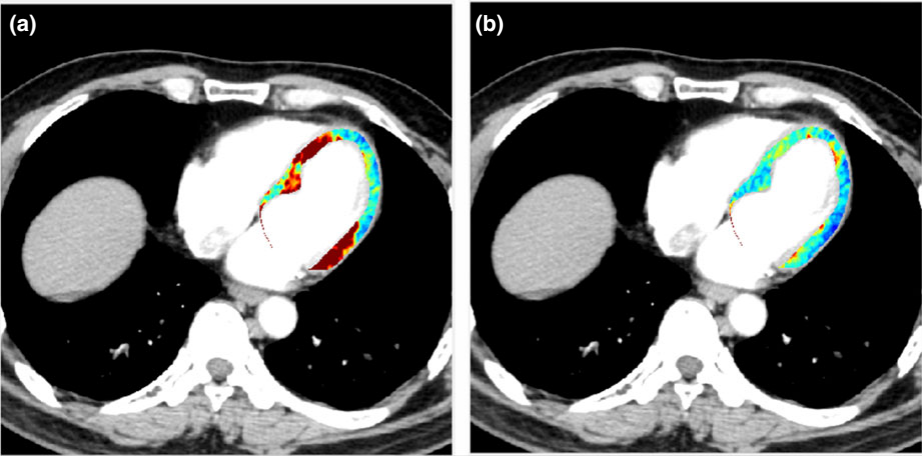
Blood flow estimation in clinical MPI‐CT, calculated from conventional (a) and ABHC (b) images. Blood flow calculated from ABHC images is more homogeneous. [Color figure can be viewed at wileyonlinelibrary.com]
